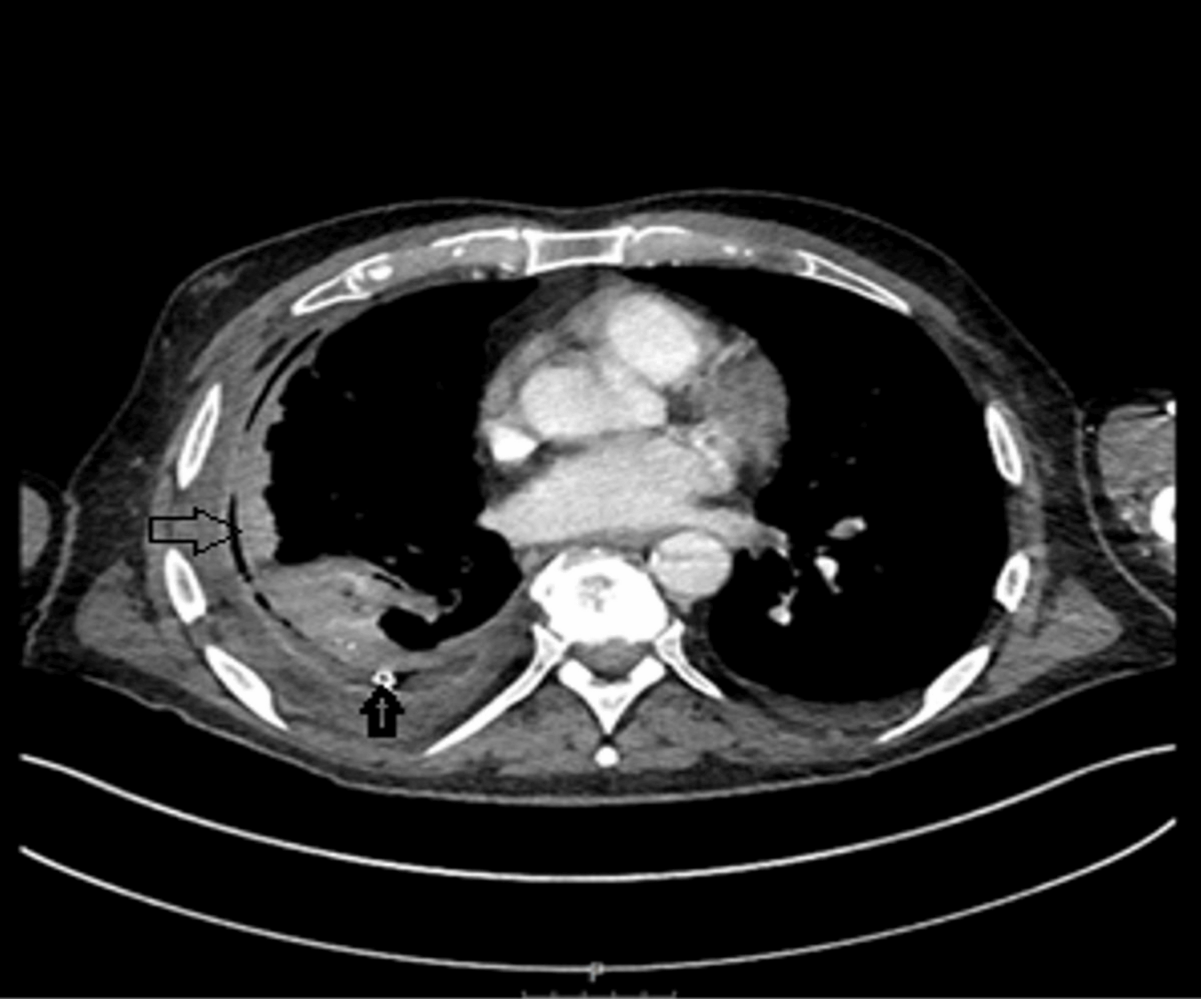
The CT scan of the chest with contrast revealed a chest drain in situ, a non-expandable lung due to a visceral pleural rind, and no evidence of mediastinal involvement.
